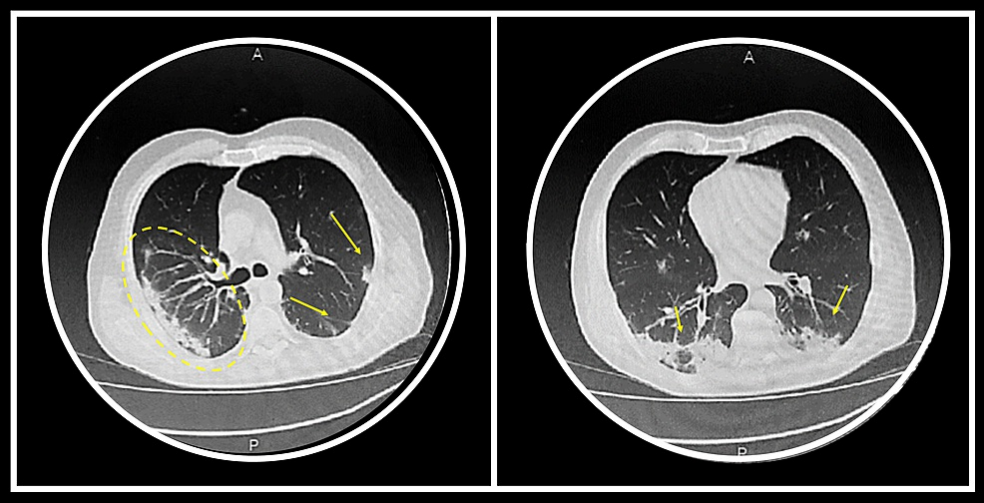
Chest CT during hospital admission Consolidations and ground-glass opacities with prevalent sub-pleural distribution are shown (yellow circle). Multiple small ground-glass areas are present in both lungs, with the same distribution (arrows). CT scans are consistent with COVID-19 interstitial pneumonia CT: computed tomography; COVID-19: coronavirus disease 2019
